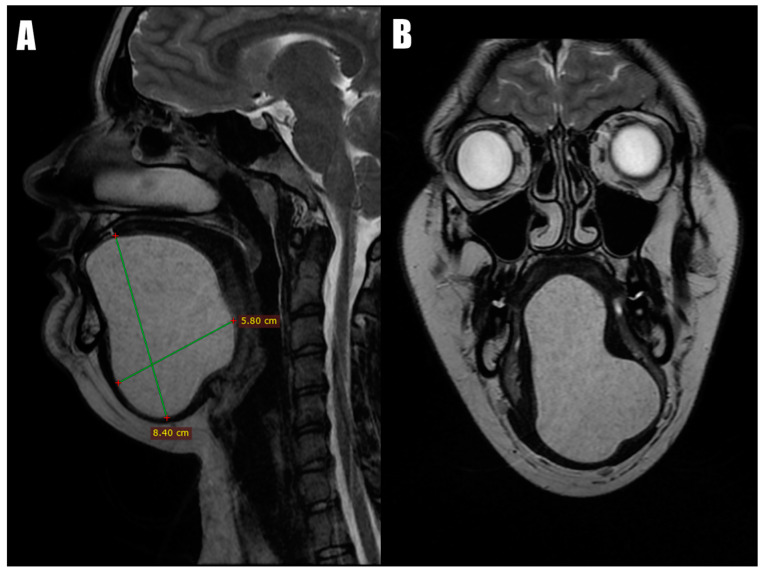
MRI scans: the coronal view (A) and the axial view (B) demonstrate that the submental and sublingual dermoid cyst extended superiorly, dividing intrinsic tongue muscles.
